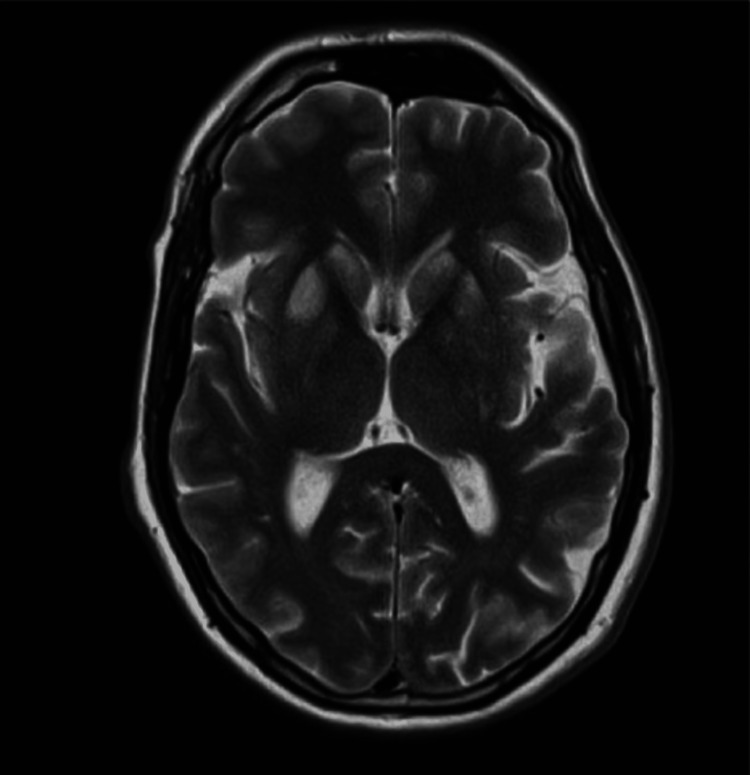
T2-weighted MRI scan showing bilateral symmetrical T2/FLAIR signal abnormality within the bilateral head of the caudate, globus pallidus, and putamen, evidence of restricted diffusion from the region of the globus pallidus on DWI FLAIR: fluid-attenuated inversion recovery; DWI: diffusion-weighted imaging
